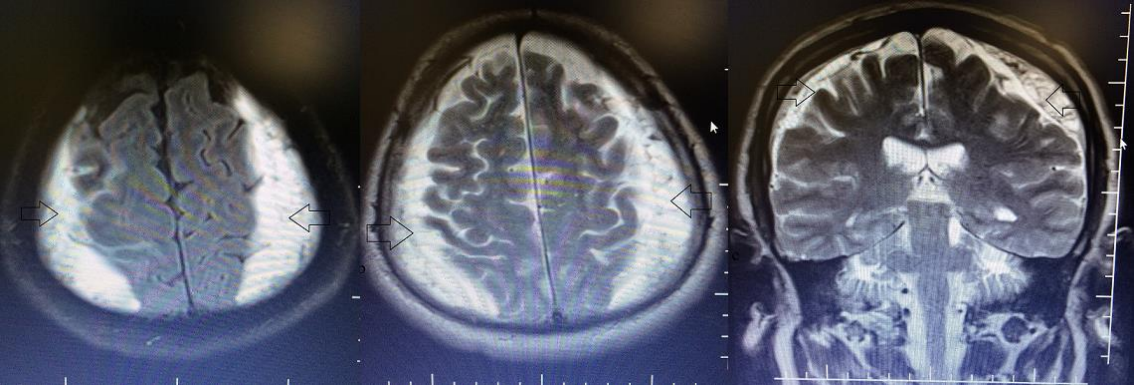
Bilateral frontotemporoparietal hyperintense images on T1 and T2-weighted axial and coronal MRI scans at initial presentation
